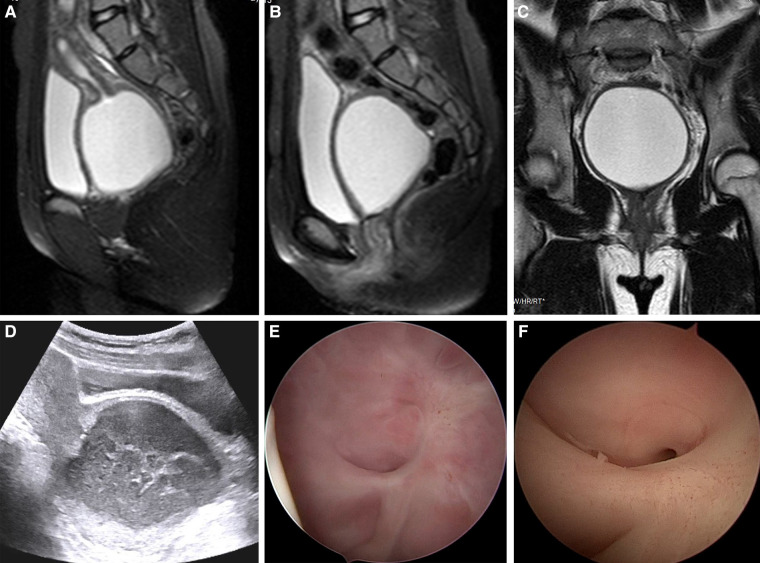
Imaging findings and outpatient hysteroscopic images of the patient. (A–C) Pelvic MRI showed a large amount of effusion in the middle and upper segments of the vagina and that the lower segment of the vagina was adhered to the bladder neck. (D) Pelvic ultrasound showed hydrocolpos (range, approximately 7.5 cm × 7.2 cm × 6.5 cm) with a flocculent echo. (E,F) Outpatient hysteroscopy revealed a small hole at the top of the vagina, which was blocked by a transverse fold, and the cervix was not observable.
